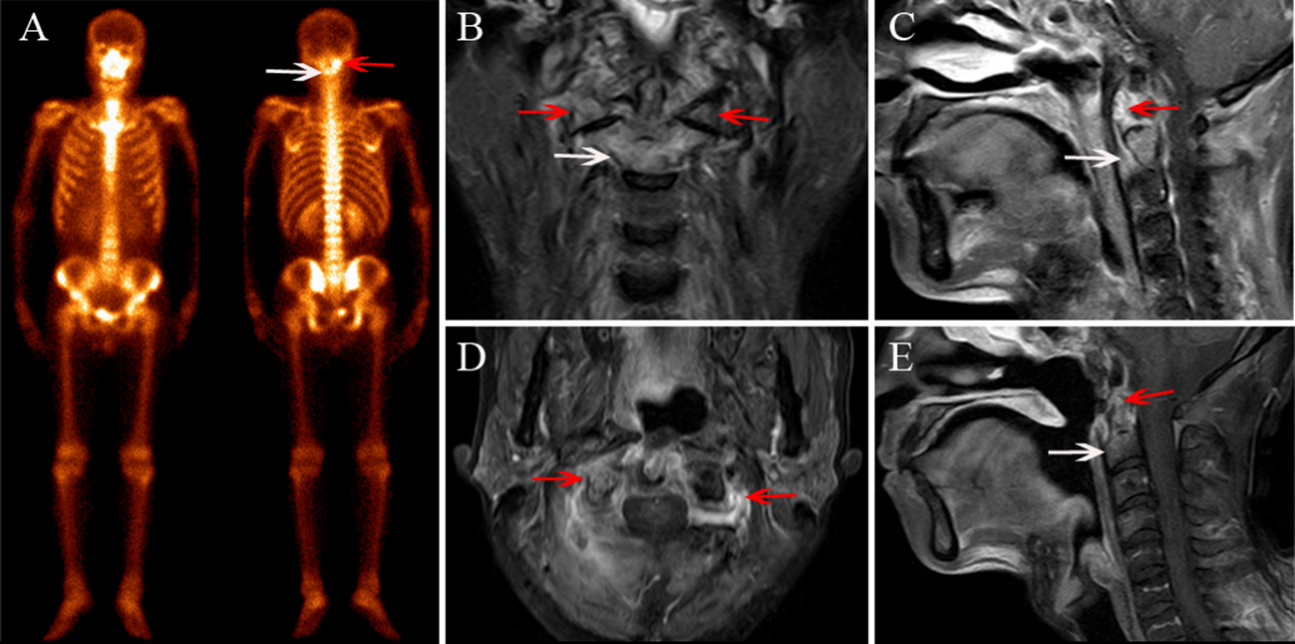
A 56-year-old female diagnosed with cervical spine ORN after RT for NPC. (A) BS shows increased radiotracer uptake in C1 (red arrow) and C2 (white arrow). (B) Coronal FS T2-weighted image shows hyperintensity in C2 (white arrow) and the bilateral aspect of C1 (red arrow). (C) Sagittal contrast-enhanced T1-weighted image shows marked enhancement in lesions. (D) Sagittal contrast-enhanced T1-weighted image shows paravertebral muscle edema and marked enhancement change. (E) MRI follow-up after 6 months and a sagittal contrast-enhanced T1-weighted image shows that the lesion area has shrunken and the enhancement has declined.
